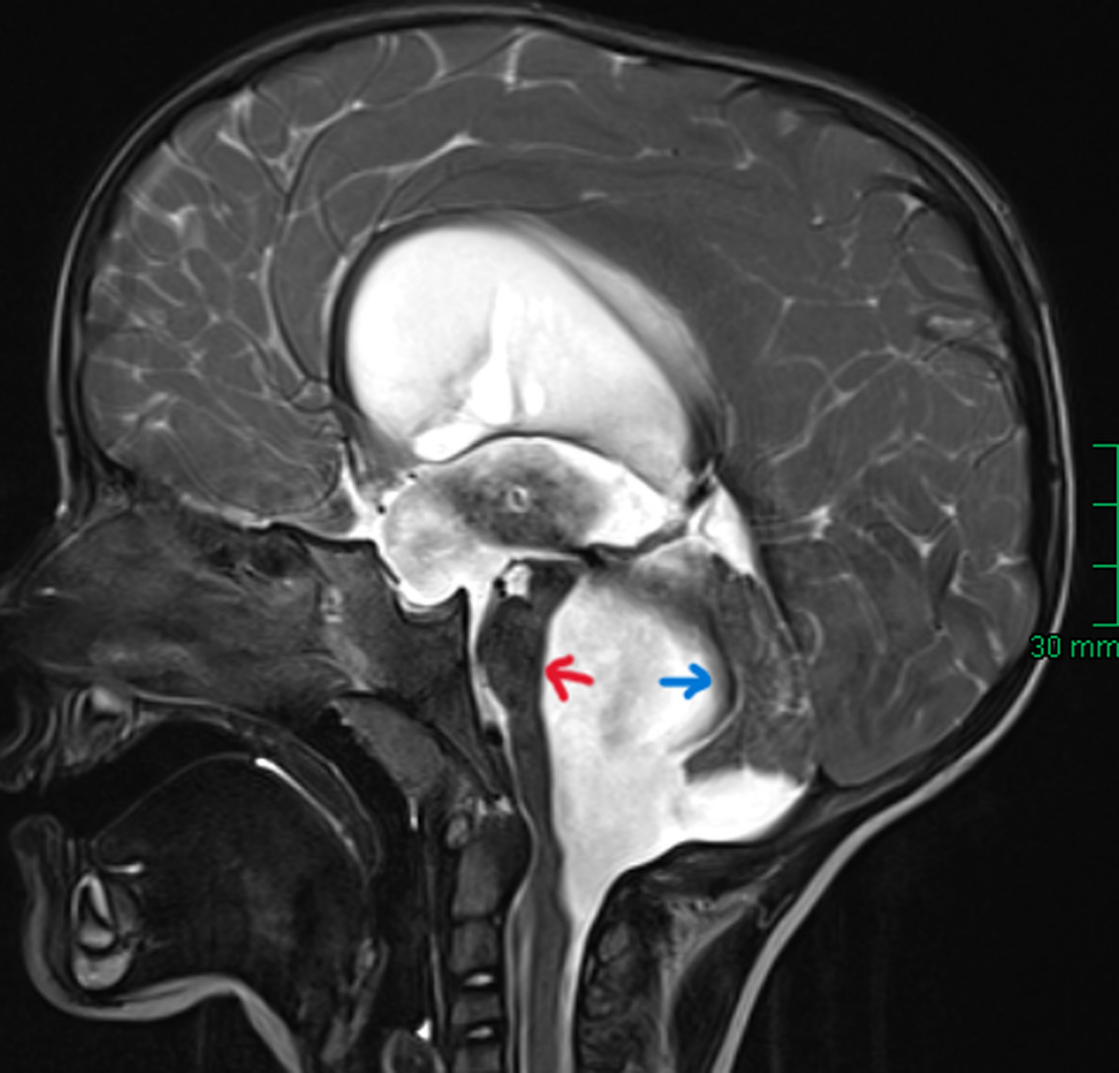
T2 sagittal MRI imaging of Case 1 showing hydrocephalus due to FVOO with a displacement of the brainstem (red arrow) and the cerebellum (blue arrow).
